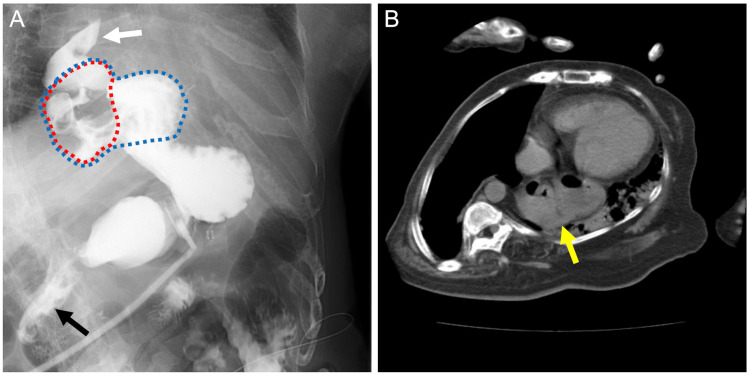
Gastrointestinal series and CT findings. (A) Upper gastrointestinal contrast imaging via a gastrostomy tube two years before this attack demonstrated the simultaneous appearance of the duodenum (black arrow) and esophagus (white arrow) due to a relapse of hiatal hernia (blue dotted circle), including prolapse of esophagofundal wrapping as a filling defect (red dotted circle). (B) CT scan at the onset of this attack indicated a hiatal hernia (yellow arrow). CT: computed tomography
